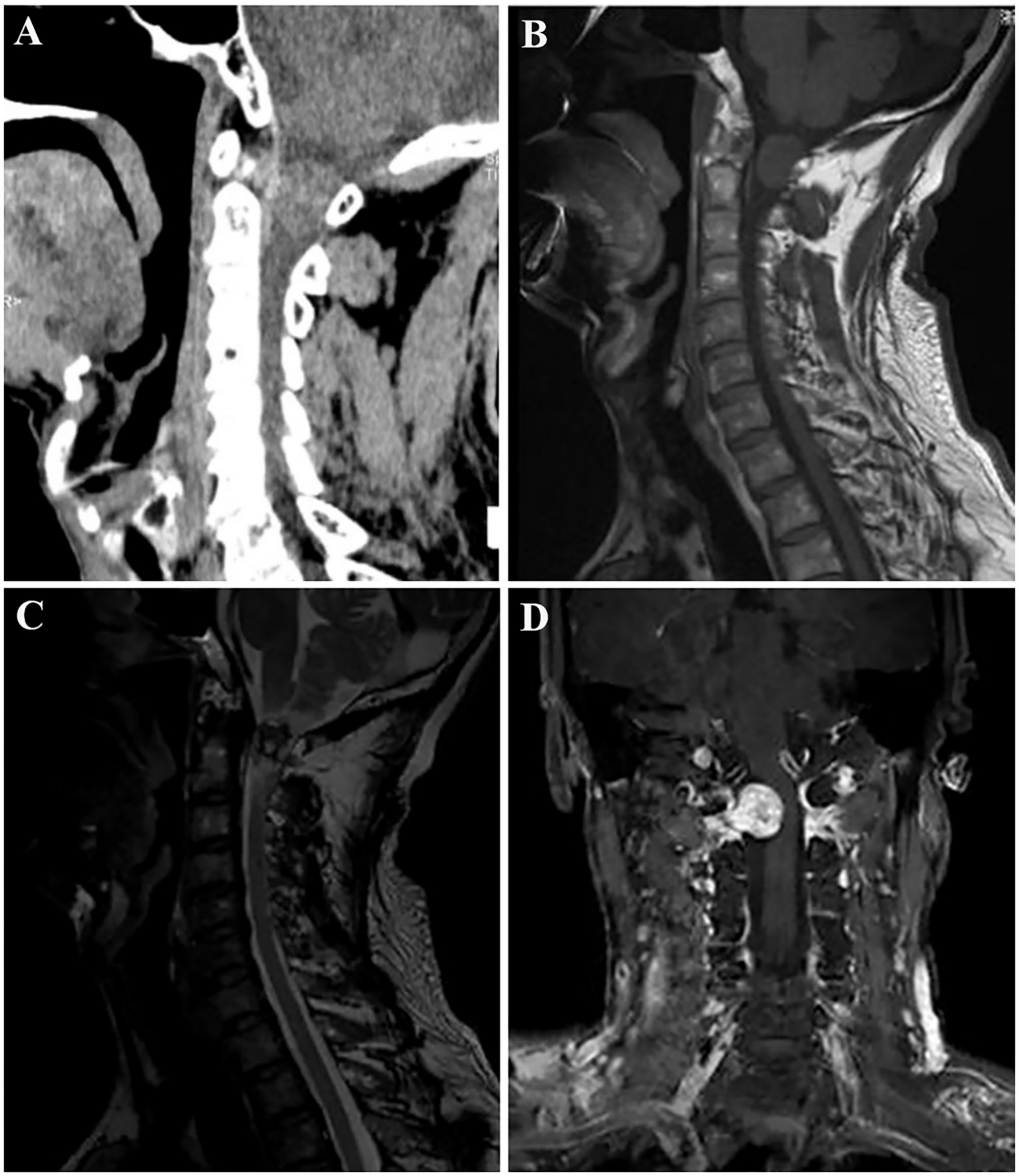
Spine computed tomography (CT) demonstrated a slightly hyperdense nodule lesion with a little spot low and high density of the C1-2 level, and well-circumscribed without obvious bone destruction (A). Contrast-enhanced magnetic resonance imaging (MRI) of the spine; the lesion was isointense on T1-weighted images (B), and hypo- to hyperintense on T2-weighted images (C). After intravenous administration of gadolinium, the tumor showed obvious peripheral enhancement, presenting a growing tendency along the right second intervertebral foramen and compressing the spinal cord to the left side (D).
